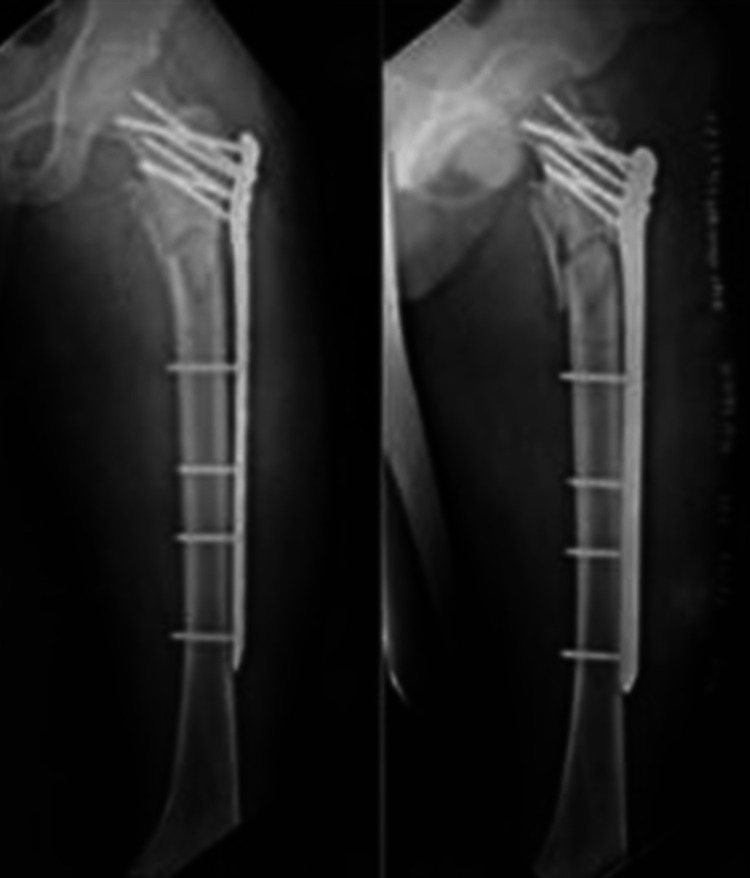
Immediate postoperative antero-posterior and lateral radiographs Fracture fixation was performed with a proximal femur locking compression plate (PF-LCP).
